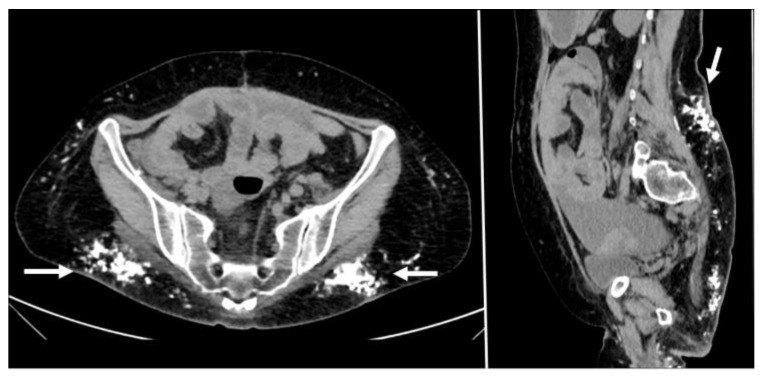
Woman with long-standing dermatomyositis. Axial and sagittal not-enhanced CT scan reveals diffuse, multiple calcifications on the subcutaneous abdominal tissue, representing the evolution of non-controlled dermatomyositis. Large peritoneal effusion is also seen.
